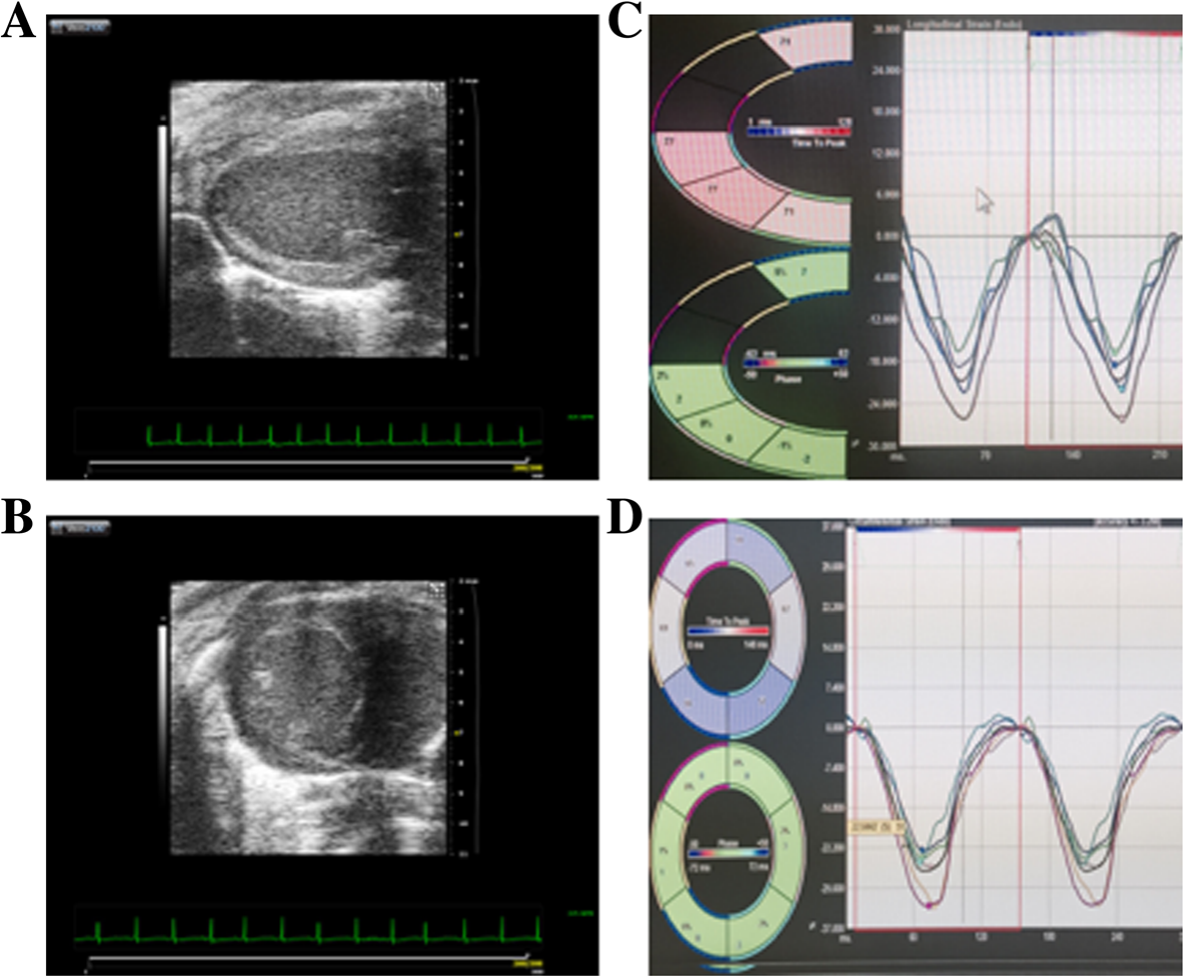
Strain analysis by echocardiogram post-processing software (VevoStrain, FUJIFILM VisualSonics Inc.). The parasternal long access view (a) was used to calculate the longitudinal strain (LS, %) (b) and the longitudinal strain rate (LSR, 1/sec). The parasternal short axis view (c) was used to calculate the circumferential strain (CS, %) (d) and circumferential strain rate (CSR, 1/sec)
